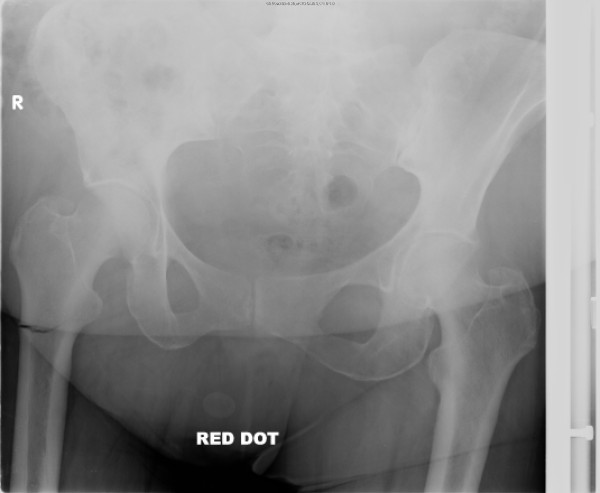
X-ray of the right hip showing an incomplete subtrochanteric fracture of right hip.
